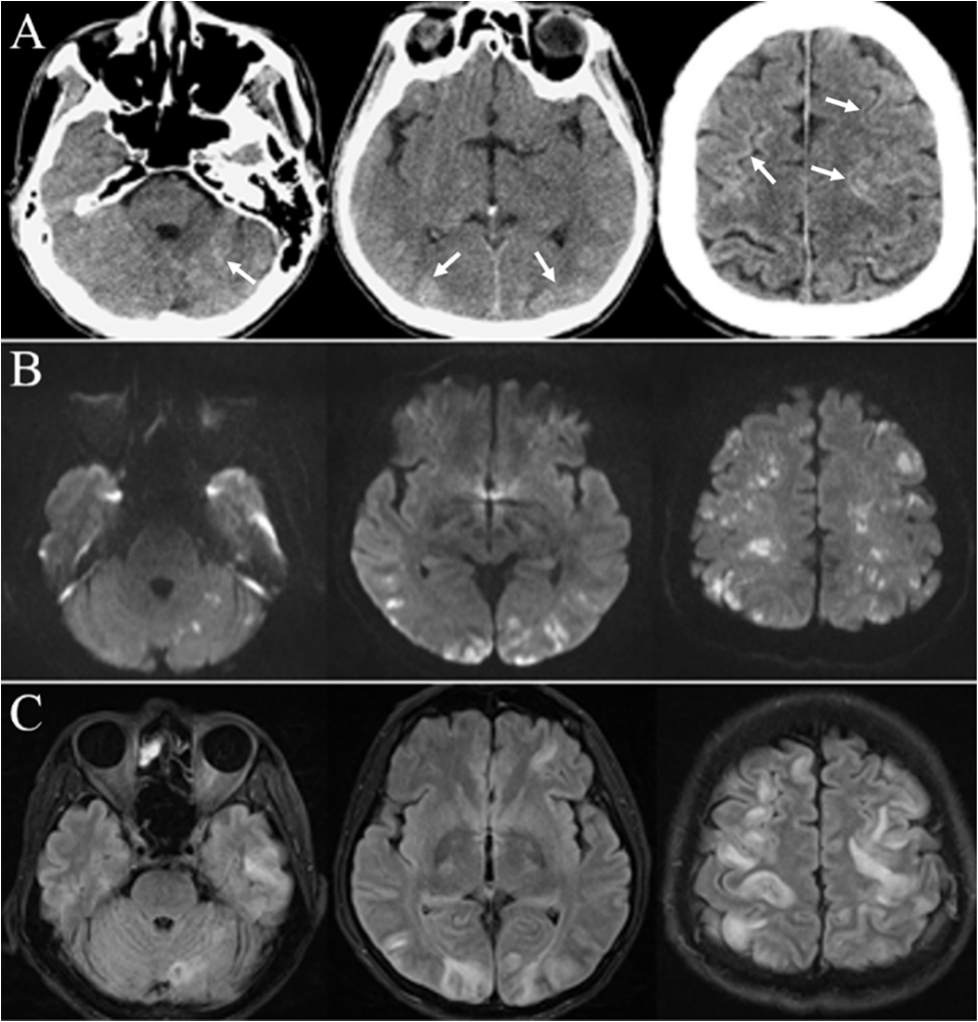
Head imaging findings in a patient with hepatocellular carcinoma who had cerebral lipiodol embolism (CLE) after transarterial chemoemobolization. Head CT and MRI were performed at 9 hours and 1 day after the symptoms, respectively. Row A: Head non-contrast CT showed disseminated high-density lesions mainly at gray matter (arrow), Row B and C: diffusion weighted imaging (DWI) and T2 fluid attenuation inversion recovery (FLAIR) of head MRI. The scans showed multiple disseminated hyperintensity/high signal lesions mainly at the gray matter of the cerebrum and cerebellum. The larger areas on FLAIR than on DWI for the same lesions indicate the existence of peri-stroke edema.
